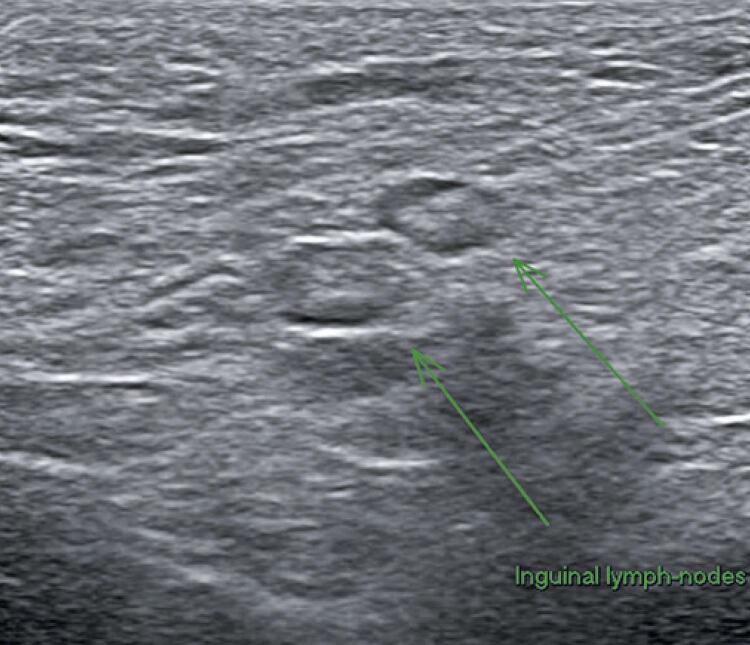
Ultrasonography demonstrating inguinal lymph nodes of a habitual size
